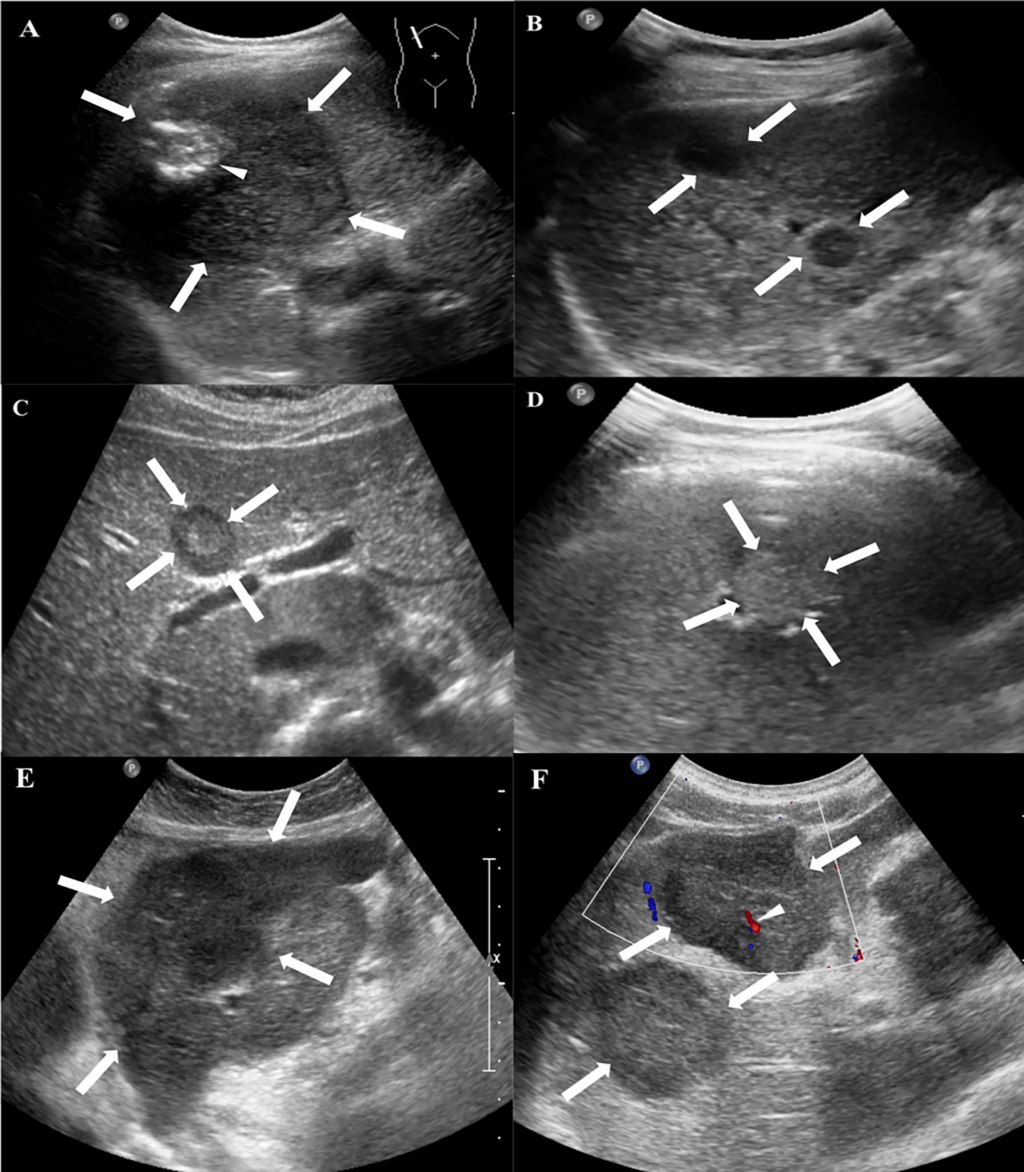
Conventional US features of HEHE in patients. (A) Grayscale ultrasound displayed a subcapsular hypoechoic mass (arrows) with well-defined margin, regular shape and focal calcification (small arrow) in a 49-year-old female. (B) Multifocal hypoechoic nodules (arrows) with a hyperechoic halo in a 48-year-old female. (C) A heterogeneous nodule with both hypo- and hyperechoic regions (arrows) in a 29-year-old female. (D) A hyperechoic nodule (arrows) with ill-defined margin in a 49-year-old male. (E) A hypoechoic mass with irregular shape and ill-defined margin in a 56-year-old female. (F) CDFI showed rod-like blood-flow signal (small arrow) in a multiple hypoechoic masses (arrows) in a 58-year-old male.
